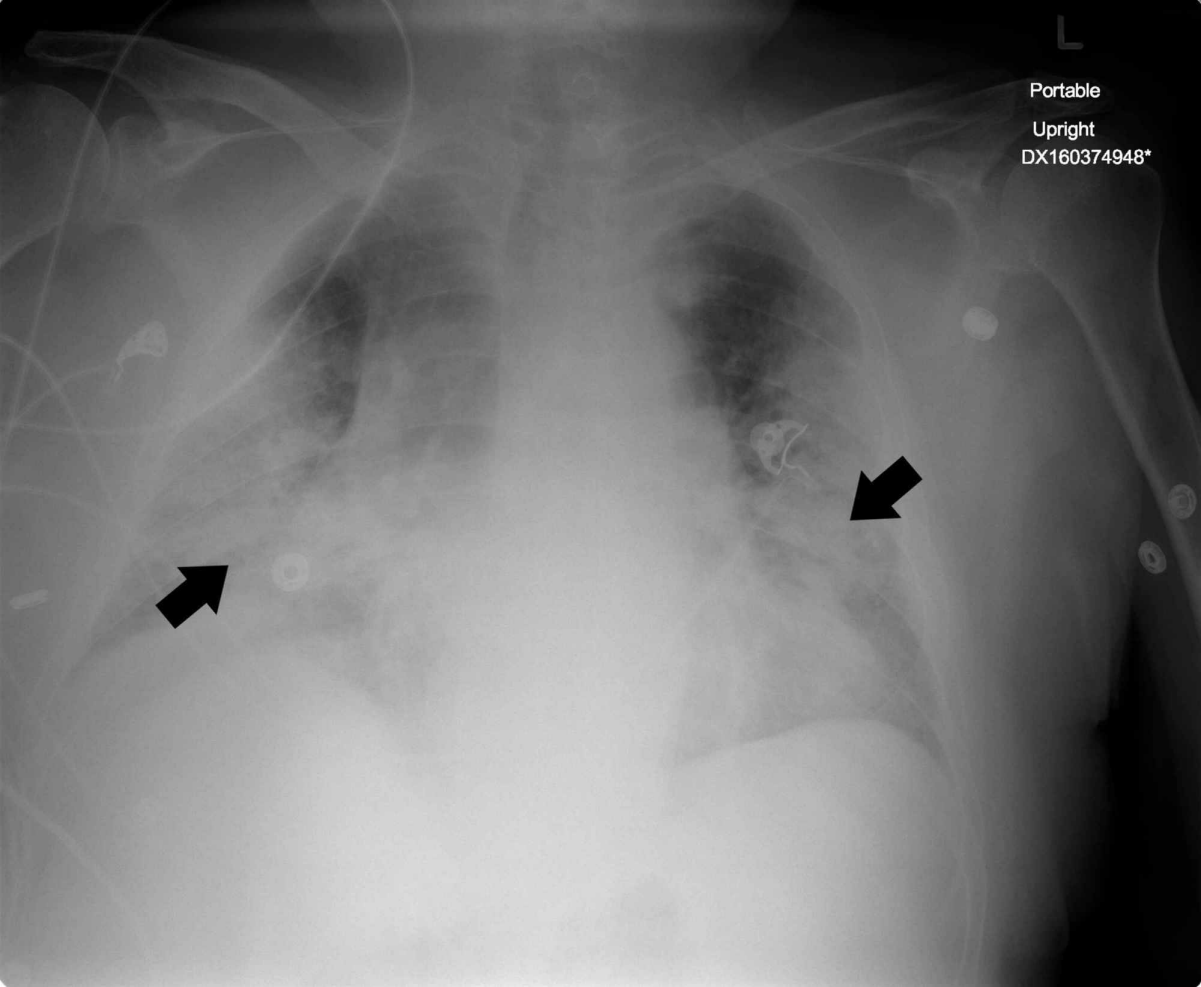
Chest radiograph 48 h following hospital admission, showing worsening bilateral pulmonary opacification (arrows).
